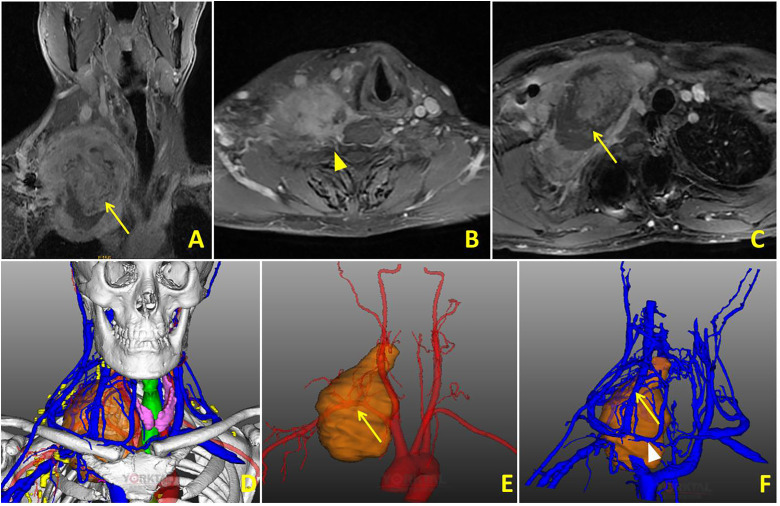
(A) Contrast-enhanced MR revealed a giant tumor extending from epiglottis level superiorly to the third intercostal space inferiorly (yellow arrow). (B) T2-weighted phase showed the tumor extending into the spinal canal through the intervertebral foramen (yellow triangle). (C) Areas of hemorrhage and necrosis within the tumor showed heterogeneous signals (yellow arrow). (D) A giant MPNST in the right thoracocervical region with severe adhesion to adjacent structures. (E) The clavicular segment of the right subclavian artery (yellow arrow) went through the tumor, with nearly normal physiological function. (F) The right internal jugular vein (yellow arrow) was pushed anteriorly, and the right subclavian vein (white triangle) had almost lost its physiological function, with collateral circulation established.
